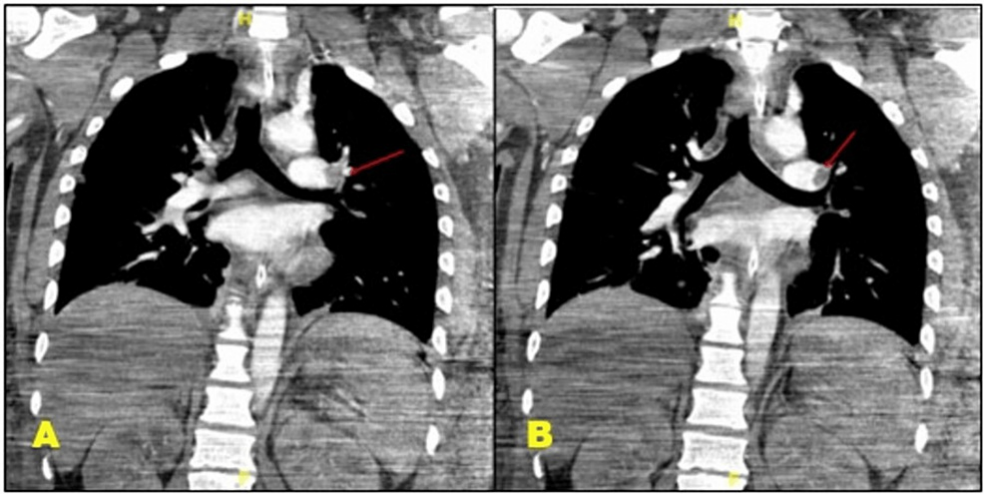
CT pulmonary angiography (coronal view) This coronal section of a CT angiogram highlights a pulmonary embolism with arrows pointing to the clot located in the pulmonary artery.
